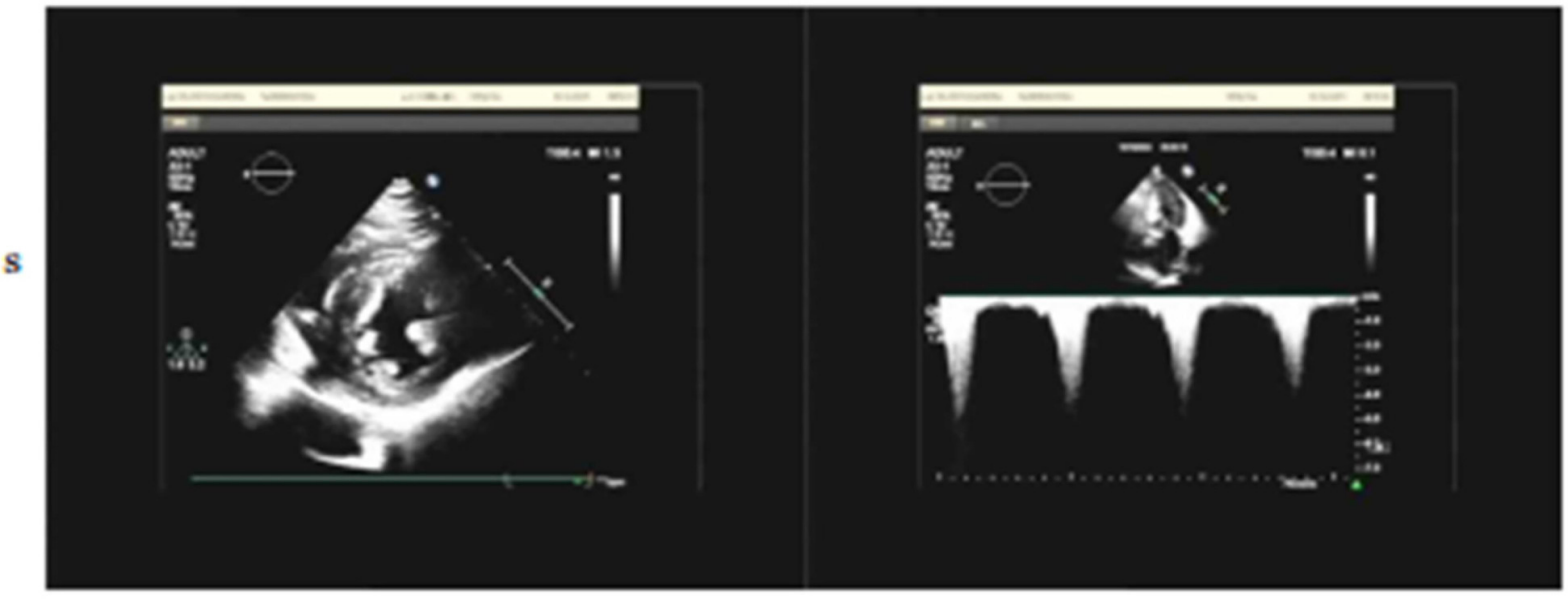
Echocardiogram upon admission.
